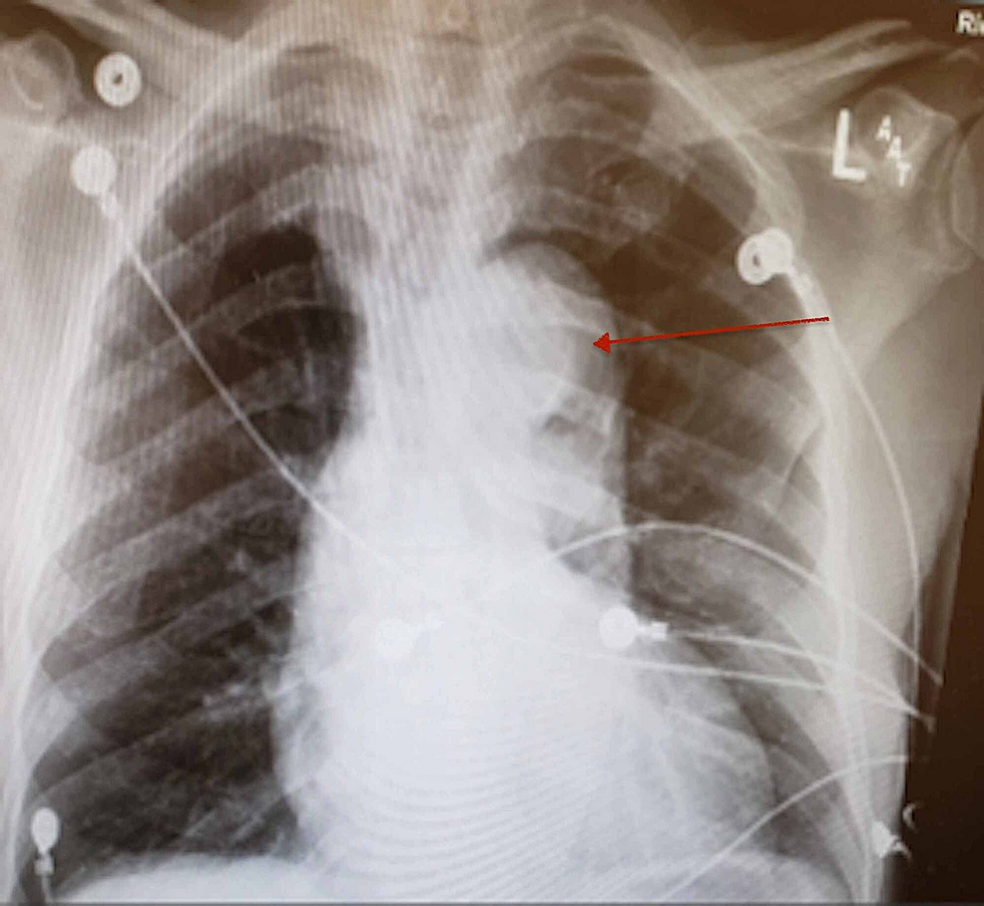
Chest x-ray: mediastinal widening with positive calcium sign (inward displacement of aortic wall calcification by more than 10 millimeters).
